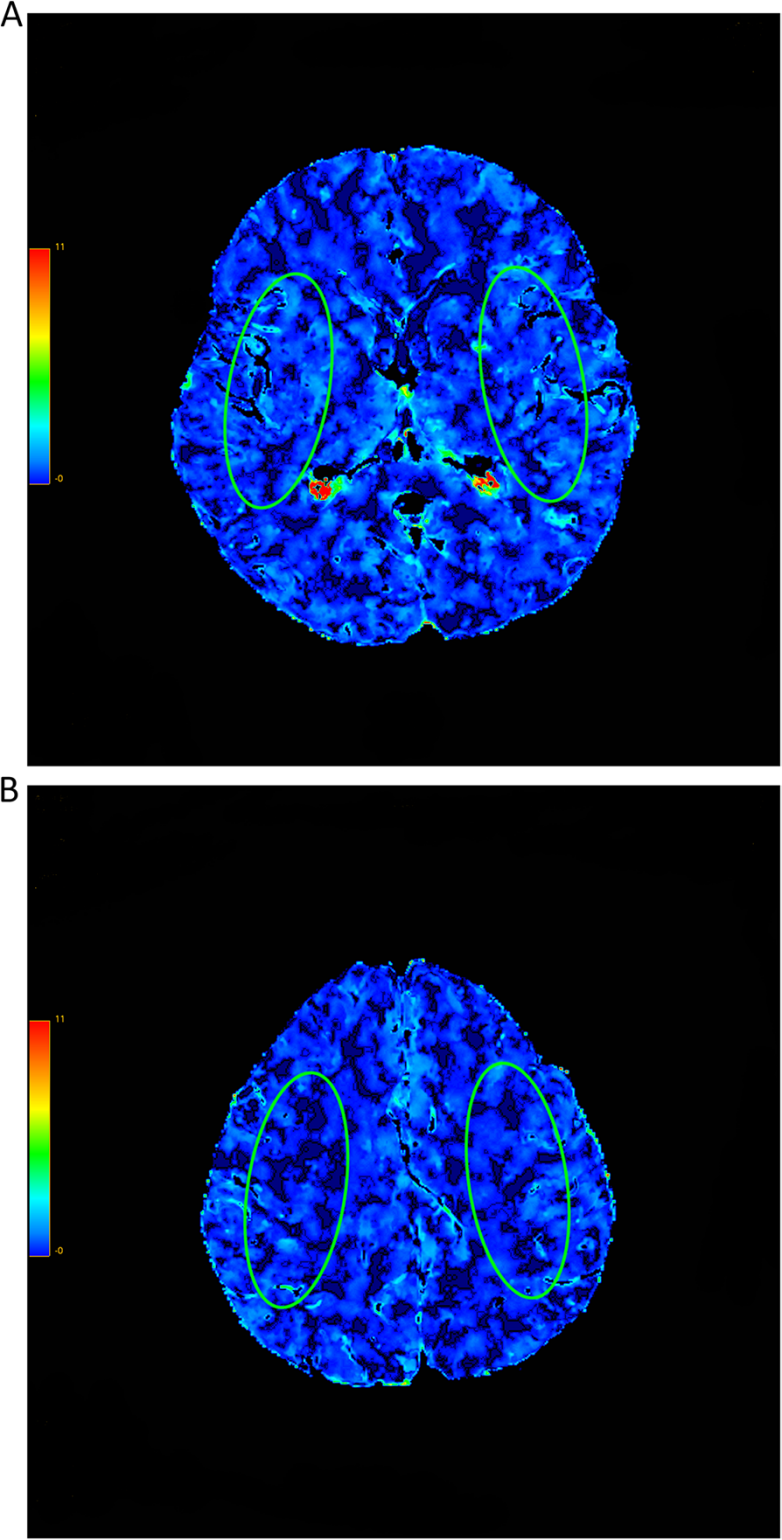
CT (computed tomography) perfusion color maps before carotid endarterectomy. Permeability surface product area (PS). Regions of interest (ROIs) placed on MCA (middle cerebral artery) territories. A The first slice of all CTP and B the last slice of all CTP
